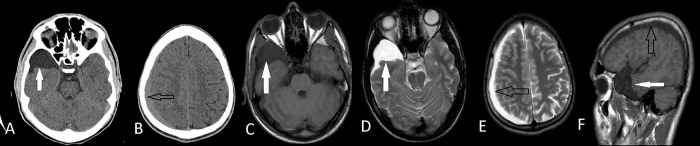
Axial CT images of right middle fossa arachnoid cyst (A) (white arrows) and ipsilateral subdural hematoma (B) (black arrows) of the Case 3. Axial T1W MRI(C), T2W MRI (D,E) and sagittal MRI (F) images shows that they are apart from each other. He had conservative treatment with a complete recovery.
